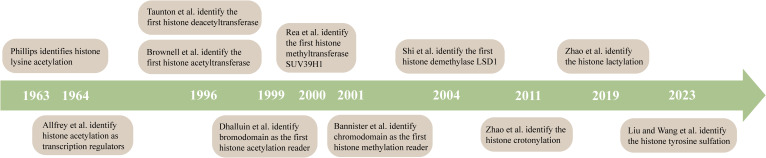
A timeline for studying histone modifications. Phillips identifies histone lysine acetylation (111). Allfrey et al. identify histone acetylation as transcription regulators (112). Taunton et al. identify the first histone deacetyltransferase (113). Brownell et al. identify the first histone acetyltransferase (114). Dhalluin et al. identify bromodomain as the first histone acetylation reader (115). Rea et al. identify the first histone methyltransferase SUV39H1 (116). Bannister et al. identify chromodomain as the first histone methylation reader (117). Shi et al. identify the first histone demethylase LSD1 (118). Zhao et al. identify the histone crotonylation (119). Zhao et al. identify the histone lactylation (120).
